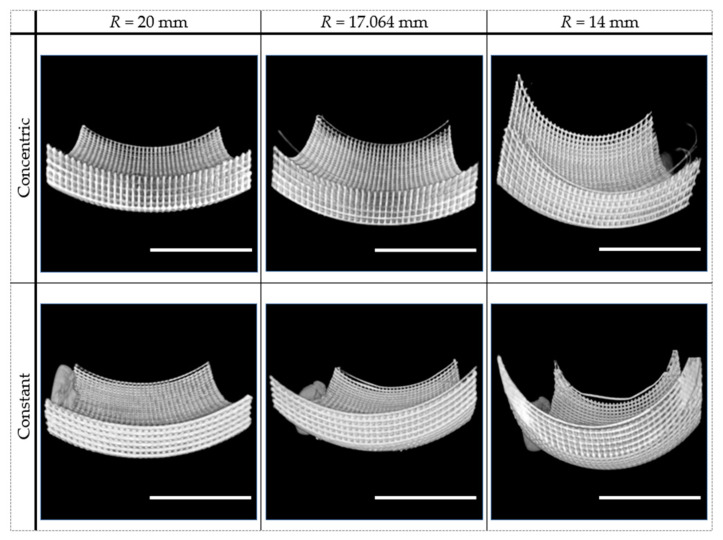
Representation of the μ-CT 3D reconstructions of the curved scaffolds using both design strategies, “concentric radius” and “constant radius”. The chosen radii (*R*) correspond to the determined limit of printability (17.064 mm) and values over (20 mm) and below (14 mm) that limit (scale bars: 1 cm).
